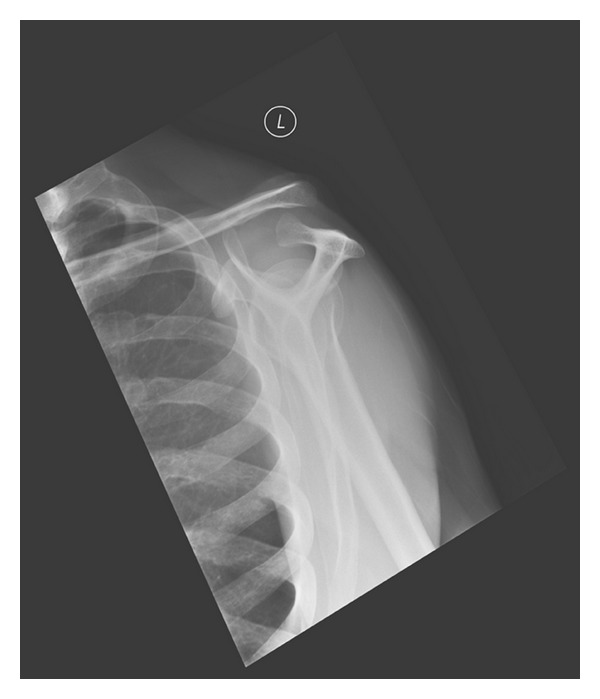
Y radiograph of the left shoulder taken 3 weeks after initial injury showing the dislocated acromioclavicular joint.
